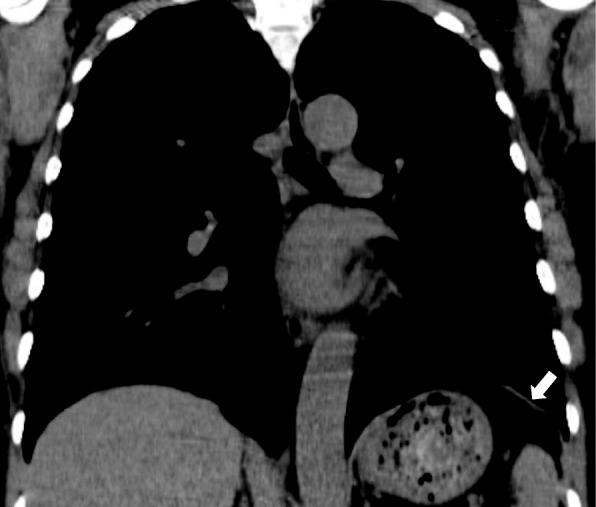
Coronal image with non-contrast chest CT scan shows a focal non-calcified plaque on the left hemidiaphragm
